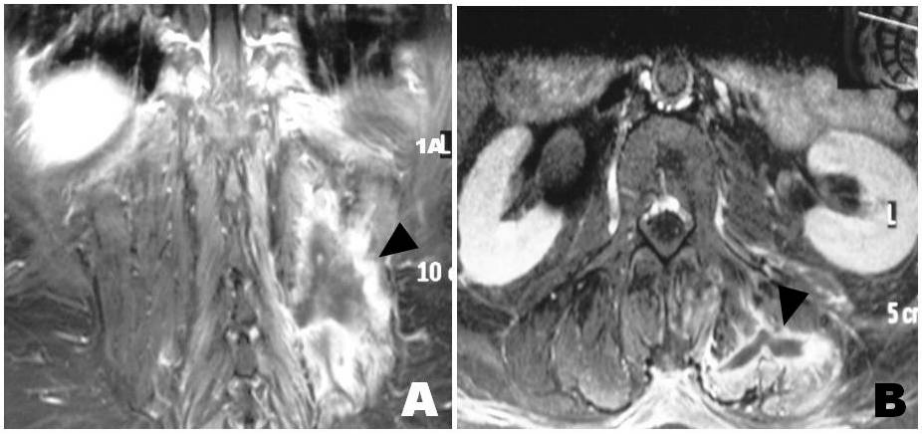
Dorso-lumbar spine magnetic resonance imaging, (A) coronal and (B) axial post gadolinium fat saturated T1: left major para-vertebral muscle with liquid collection (necrosis) and marked contrast-enhancement (inflammation) (arrowheads).
